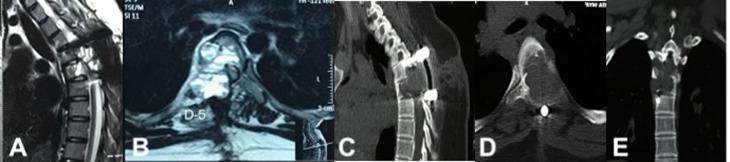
Post-operative day 2 MRI showing surgical mass compressing dorso-laterally from right (A, B), CT scan depicting the extent of bony destruction (C–E) with pedicle screw fixation and excision of ABC.
